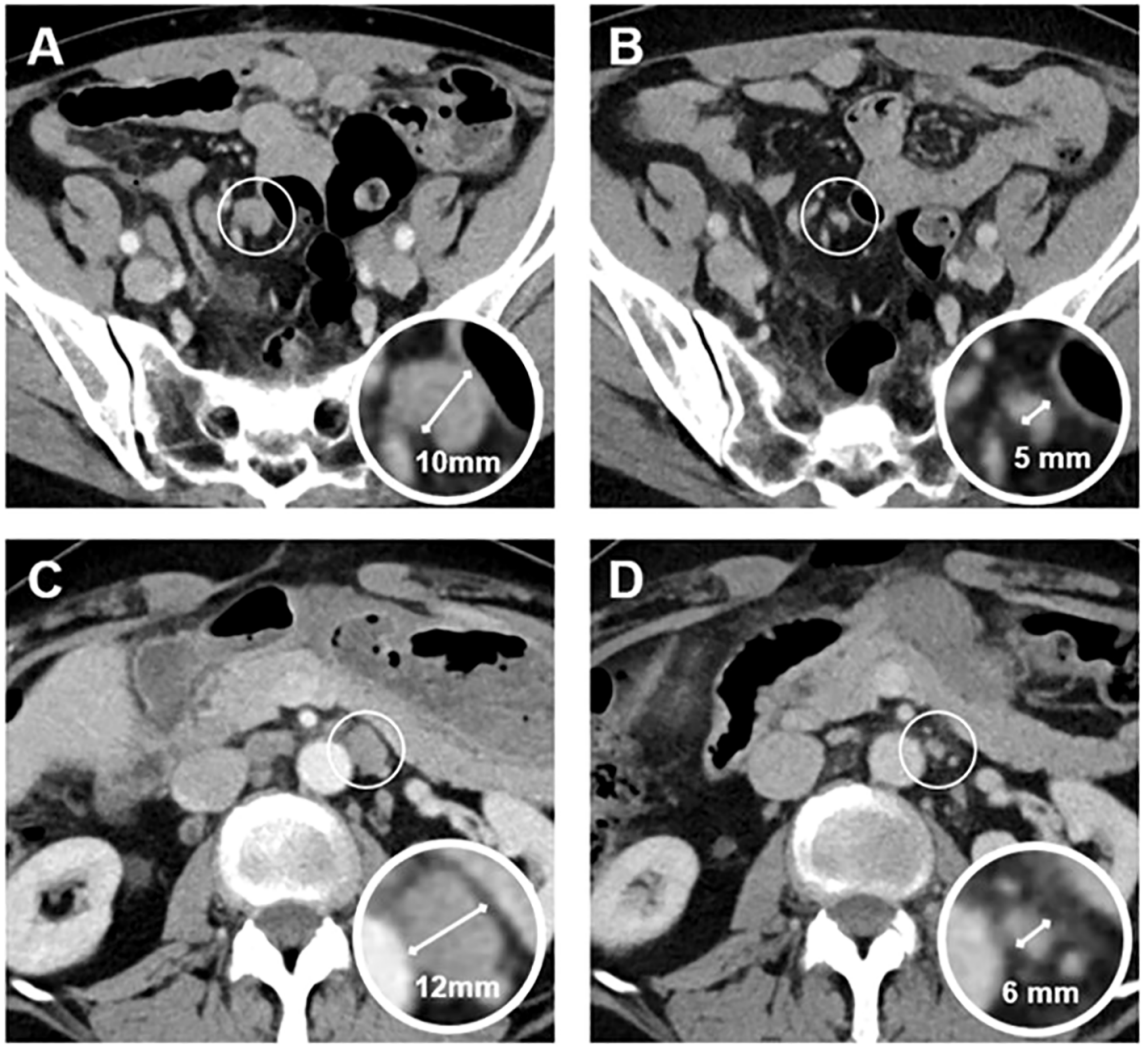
Contrast-enhanced axial abdominal CT images of a 59-year-old woman with platinum-resistant ovarian cancer, acquired before (A, C) and after (B, D) six cycles of treatment with Mirvetuximab-Soravtansin. The images and corresponding magnified views demonstrate mesenteric (A, B) and paraaortic (C, D) lymph node metastases. Post-treatment images (B, D) show normalization of lymph node size, as indicated by measurements.
